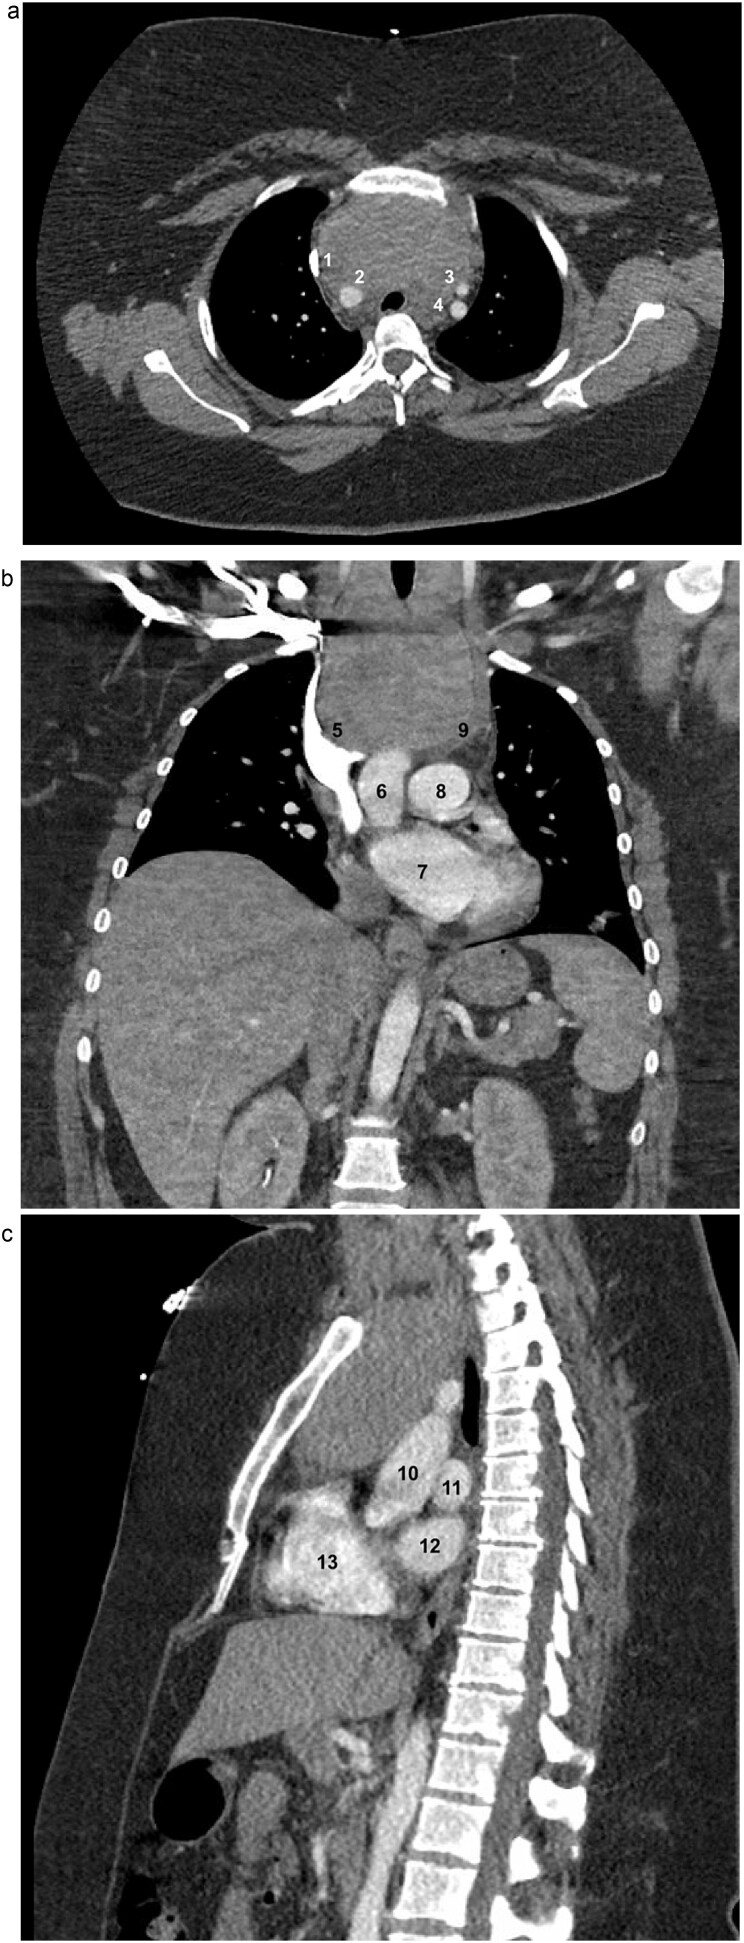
Axial (a), coronal (b), and sagittal (c) contrast-enhanced CT images of the chest demonstrating marked thyromegaly with retrosternal and intrathoracic extension (craniocaudal dimension up to 14 cm), resulting in tracheal narrowing at the thoracic inlet. In the axial view, the (1) superior vena cava (SVC), (2) brachiocephalic artery, (3) left common carotid artery, and (4) left subclavian artery are identified. In the coronal view, the (5) SVC, (6) ascending aorta, (7) left atrium (LA), (8) main pulmonary artery, and (9) brachiocephalic vein are identified. In the sagittal view, the (10) aorta, (11) left pulmonary artery, (12) LA, and (13) right ventricle are identified.
